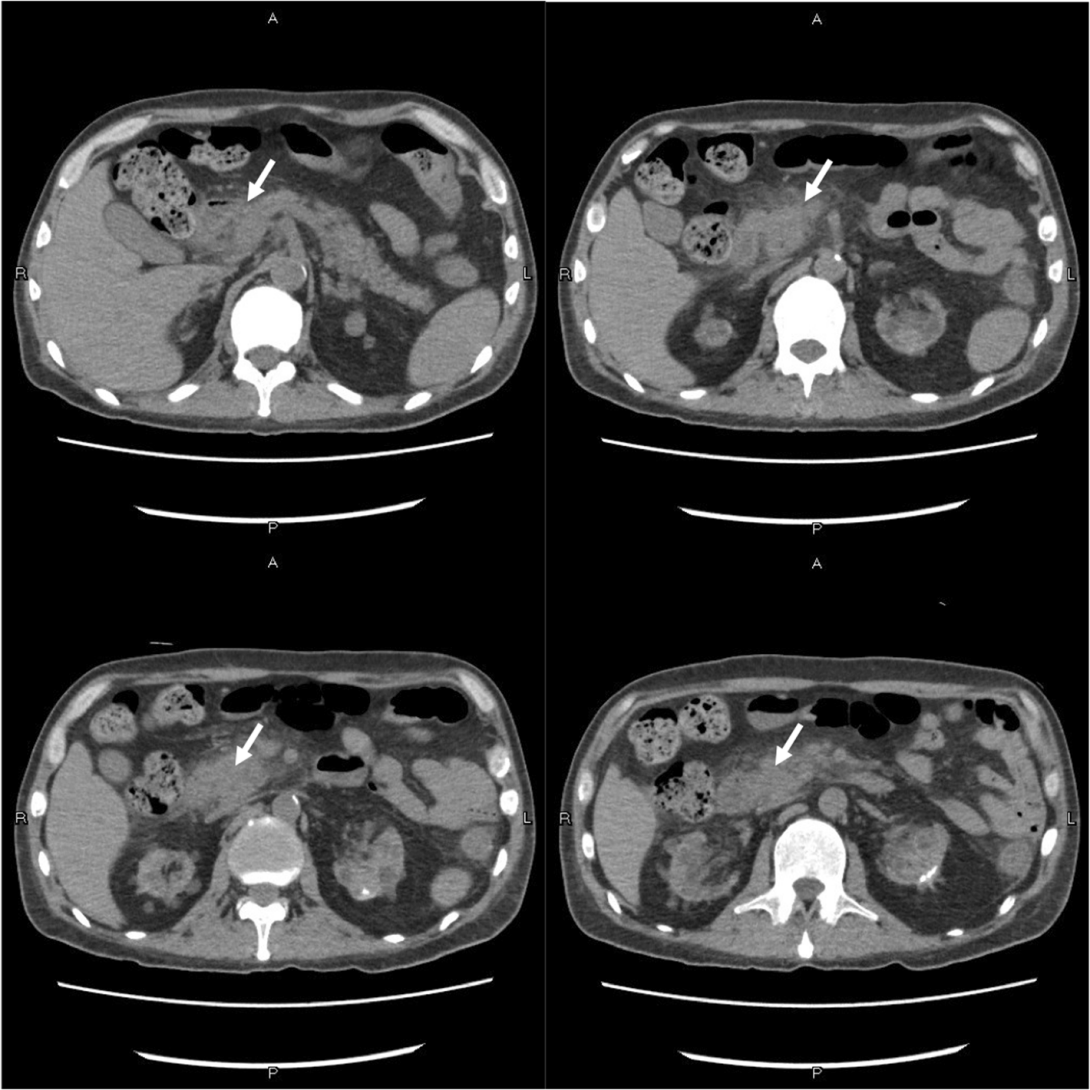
Abdominal computed tomography scan showed swelling pancreatic head and peripancreatic inflammation (white arrow)
